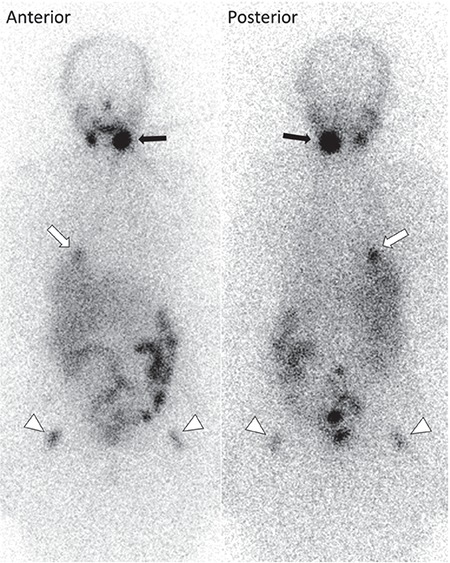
After the diagnosis of metastases, 200 mCi I-131 was applied. Tg was 77.2 ng/mL while TSH was 79 μIU/mL on the day of treatment. Post-therapeutic I-131 WBS showed focal uptakes on the right lower hemi-thorax (white-arrows) and around the hips (arrow-heads) in addition to hyperactivity on the left submandibular gland (black-arrows) and physiological gastrointestinal activities. FDG-avid metastatic pre-tracheal lymph node was false-negative on I-131 WBS.
